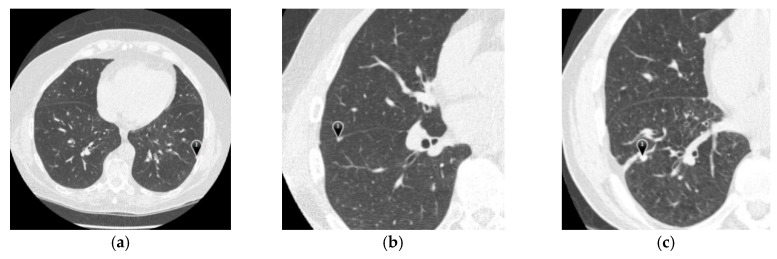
Demonstration of lung CT images from ELCAP [12]: (a) the complete CT images, (b,c) are the part of CT scans. The symbol “1” is the location of nodule.
